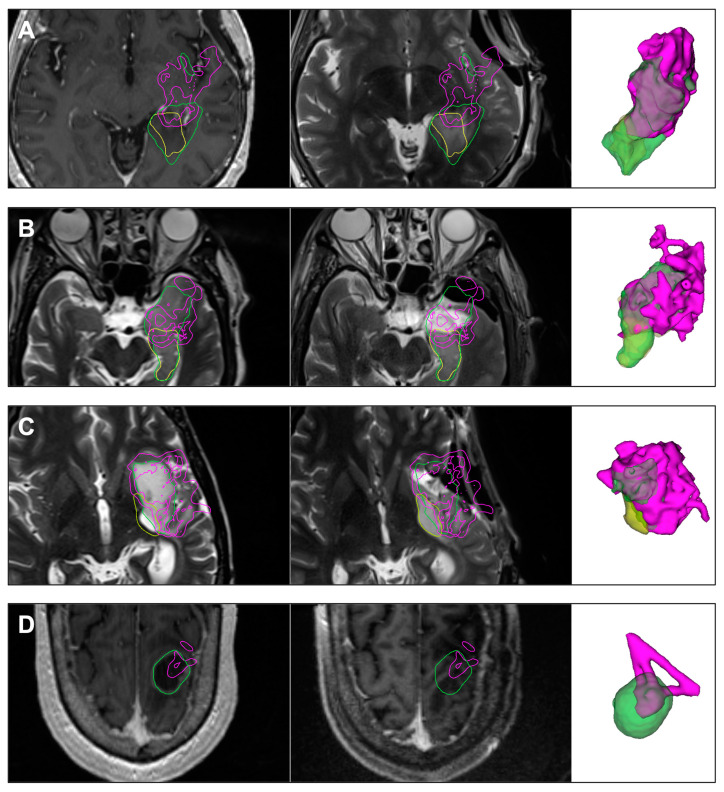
Select clinical cases. Each row shows, left to right, the preoperative MRI, intraoperative MRI, and 3D models of the tumor segmented on preoperative MRI (green), residual tumor segmented on intraoperative MRI (yellow), and the volume traced out by the tracked tool-tip positions of surgical instruments (pink). The contours of the models are also shown in the MRI images in their respective colors. In Panels A and B (Cases 12 and 36), the tracked resection cavity accurately represents the true resection cavity. In Panel C (Case 31), residual tumor volume estimation appears limited due to brain shift, but the residual location is accurate, nevertheless. In Panel D (Case 9), the tracked resection cavity does not cover the entire tumor even though there is no residual. This occurred because draining the large cystic component collapsed the resection cavity during surgery.
